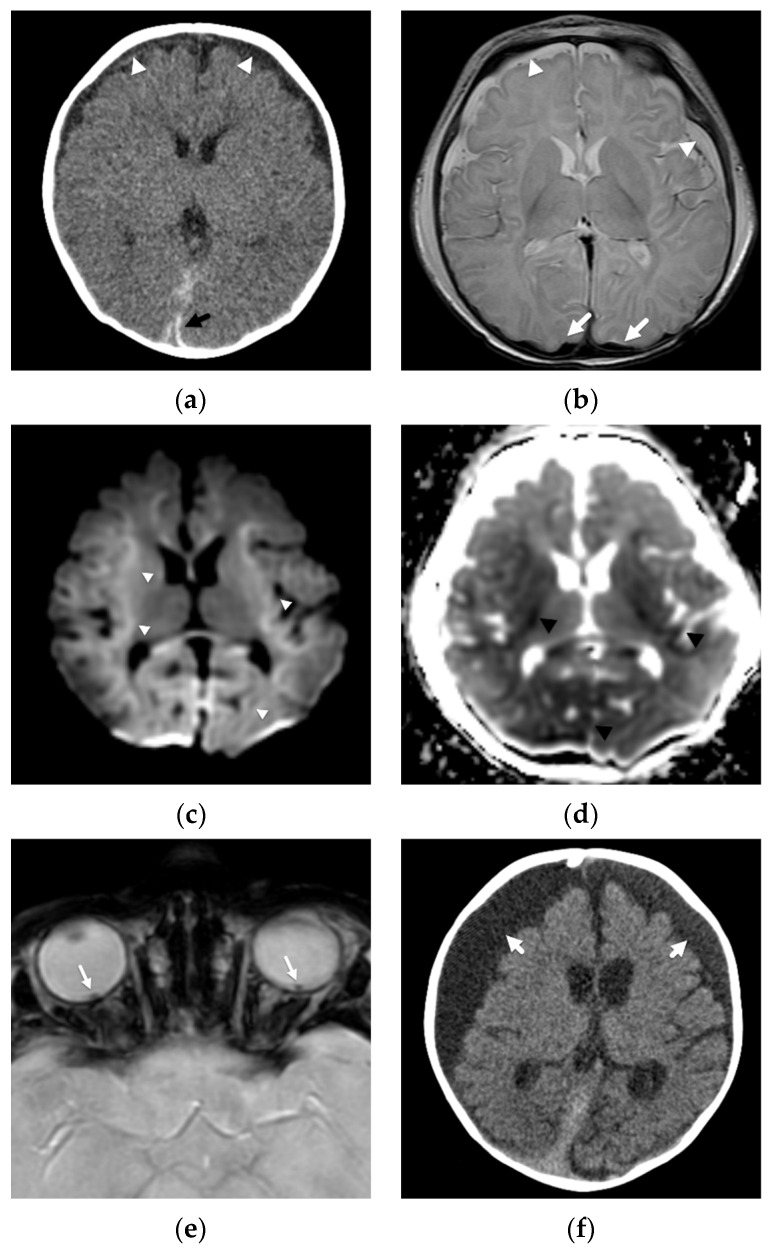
Subdural hemorrhage and subdural collections in an unresponsive 2-month-old male presenting with forehead bruising and respiratory depression. (a) Axial noncontrast head CT image demonstrates thin, high attenuation subdural hemorrhage along the posterior left occipital lobe (black arrow) and bilateral, low attenuation frontal subdural collections (white arrowheads). There is also subtle loss of gray-white matter differentiation bilaterally; (b) axial T2-weighted MR image on day 4 shows regions of decreased gray-white matter differentiation throughout both cerebral hemispheres with T2 hyperintense subdural collections (white arrowheads) and hypointense subdural hemorrhage posteriorly (white arrows); (c) axial b-1000 diffusion-weighted image demonstrates diffuse areas of abnormal, restricted diffusion (white arrowheads) in both cerebral hemispheres consistent with cytotoxic edema/hypoxic-ischemic injury; (d) apparent diffusion coefficient image shows corresponding regions of diffusion restriction predominantly in cortical and subcortical regions of both cerebral hemispheres (black arrowheads); (e) axial susceptibility-weighted image shows small, bilateral retinal hemorrhages (white arrows), confirmed clinically; (f) axial CT image at 2 month follow-up show diffuse brain parenchymal volume loss with now large, bilateral subdural collections (white arrows).
